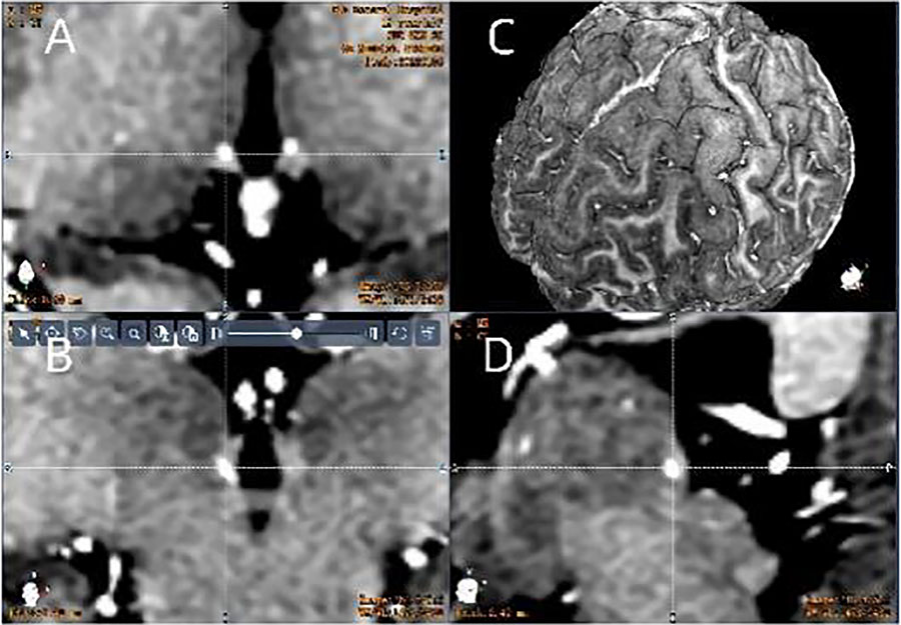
Achieved electrode positions in the lateral habenula nucleus (LHb). Fusion of postoperative CT (electrode artifacts white dots) with preoperative functional MRI. The Hb is visible (with the intersection of the white dotted line indicating the center of the left Hb) in 3D T1‐weighted MR images. (A) Axial; (B) coronal; (C) whole‐brain imaging; (D) sagittal.
